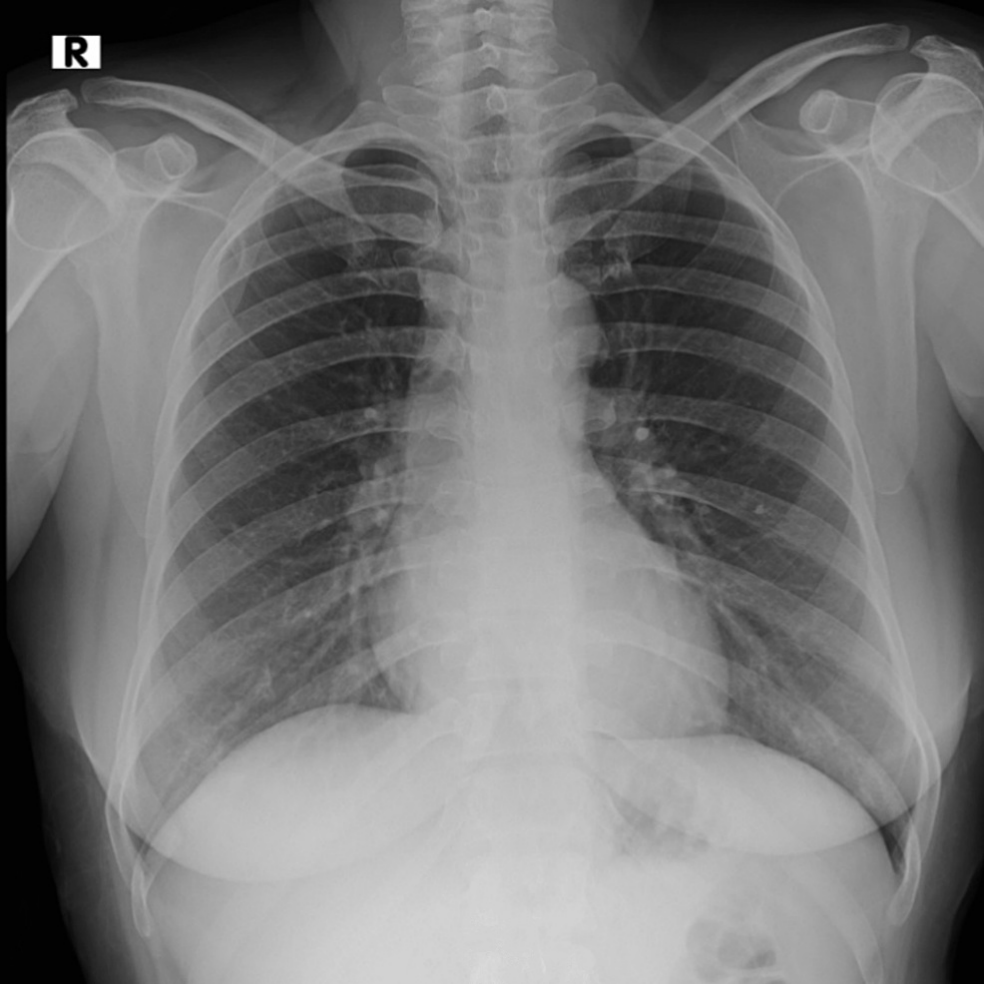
Preoperative chest X-ray showing normal bronchovascular markings
